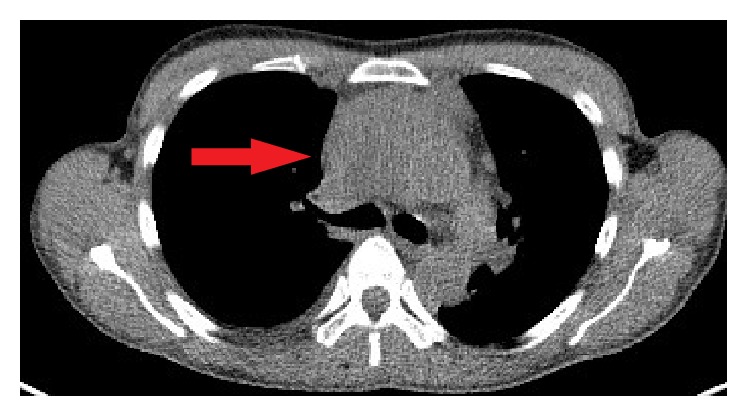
Axial CT demonstrating large anterior mediastinal sarcoma with involvement of the right atrium.
